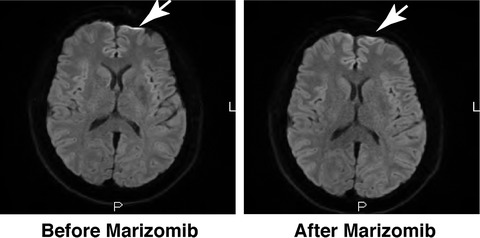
MRI brain showed CNS infiltration with leptomeningeal enhancement (before Marizomib, arrow) and resolution of the leptomeningeal enhancement post marizomib administration
